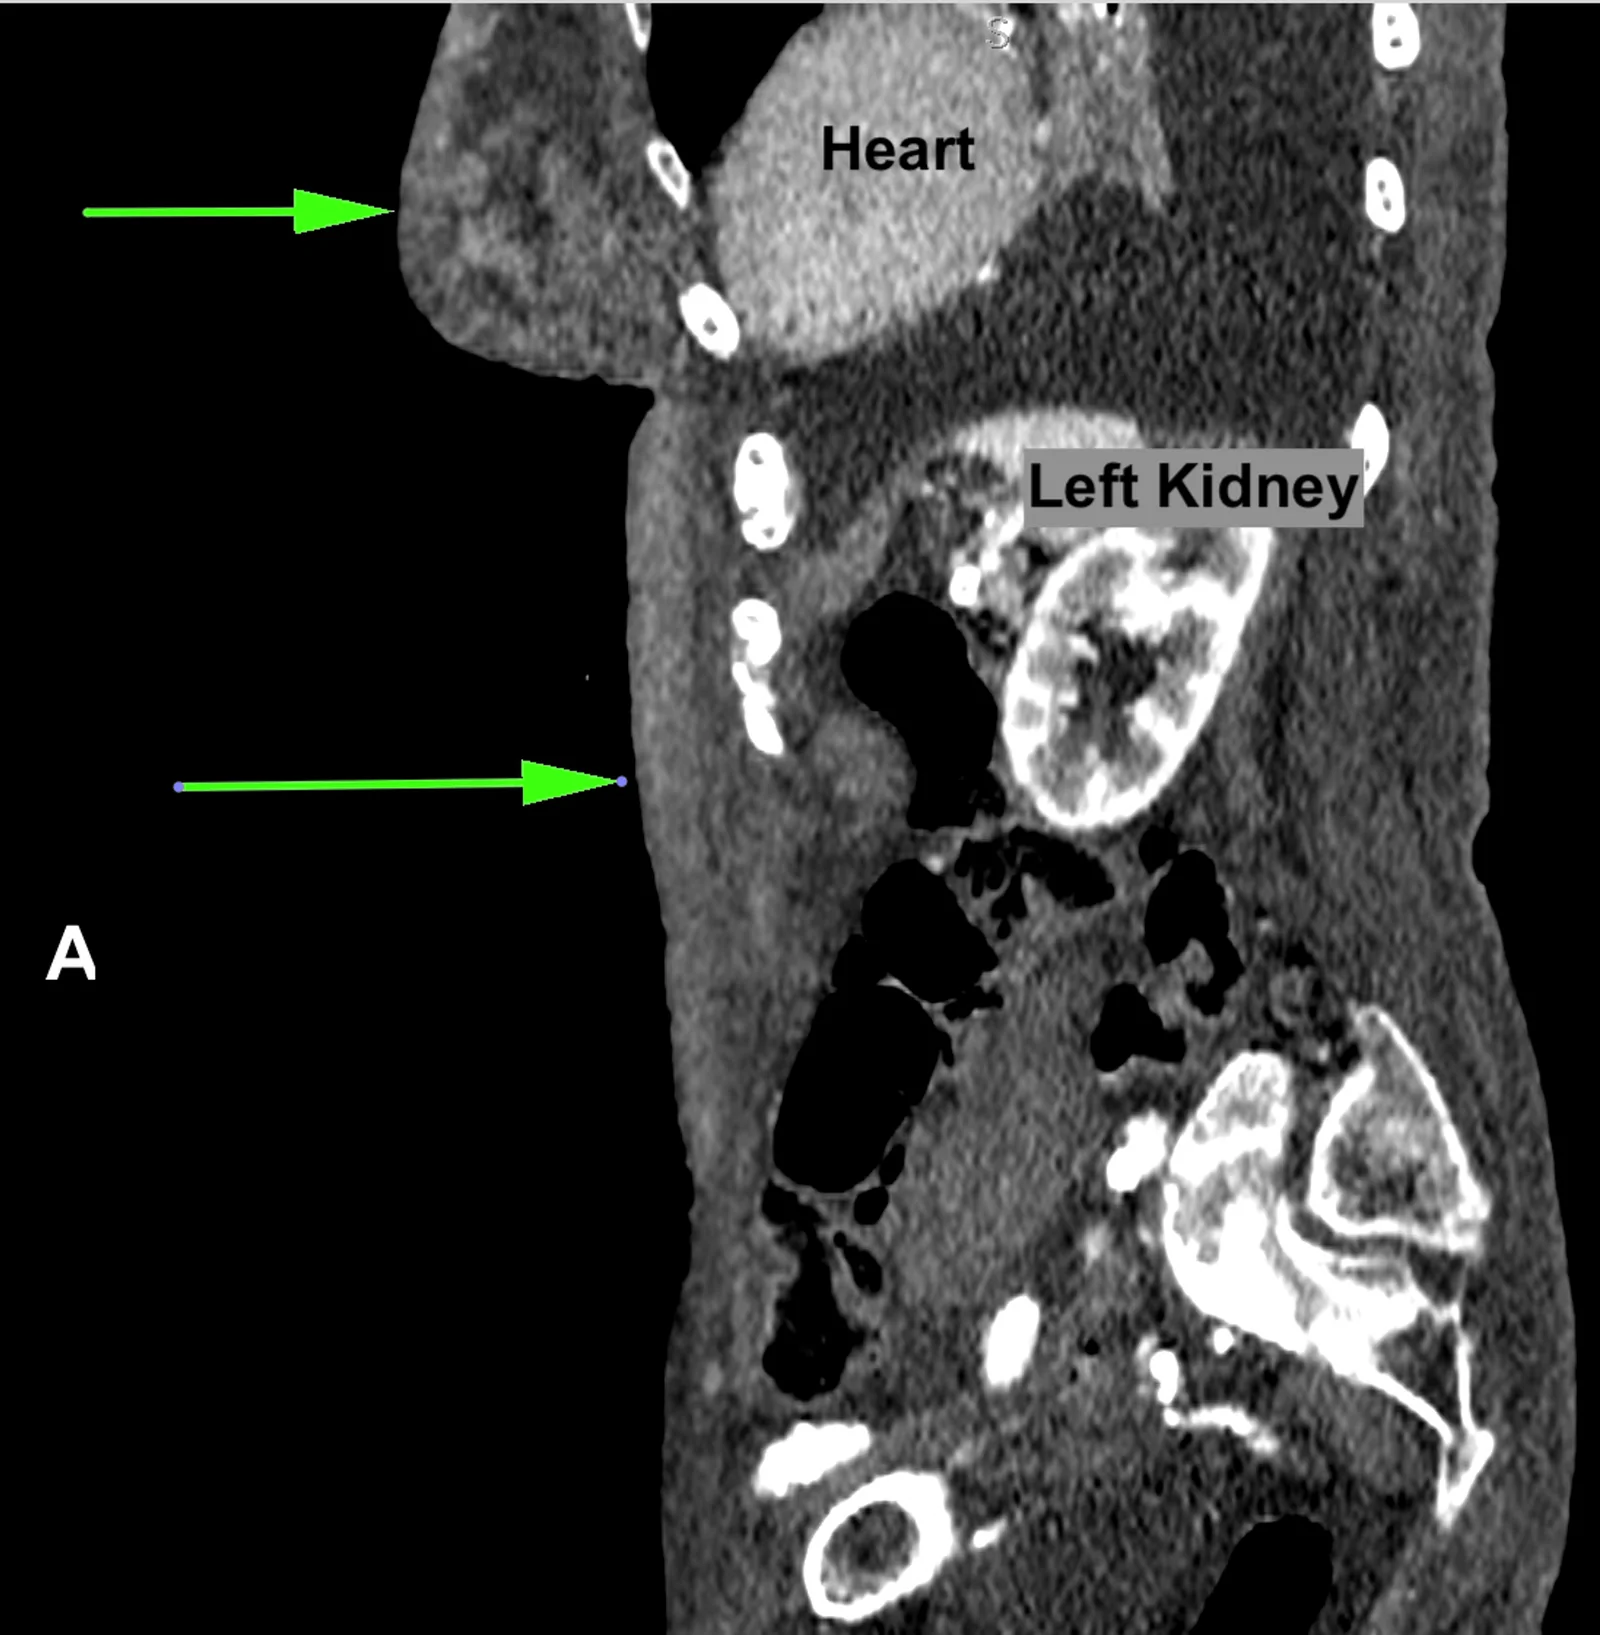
Contrast-enhanced CT scan (sagittal view) of lower chest, abdomen, and upper pelvis. Irregular skin thickening (green arrow) extending from the left breast to the lower abdomen is demonstrated. The soft-tissue densities can also be seen within the visible breast. The heart and left kidney are labelled for reference. A, anterior of the patient; CT, computed tomography
